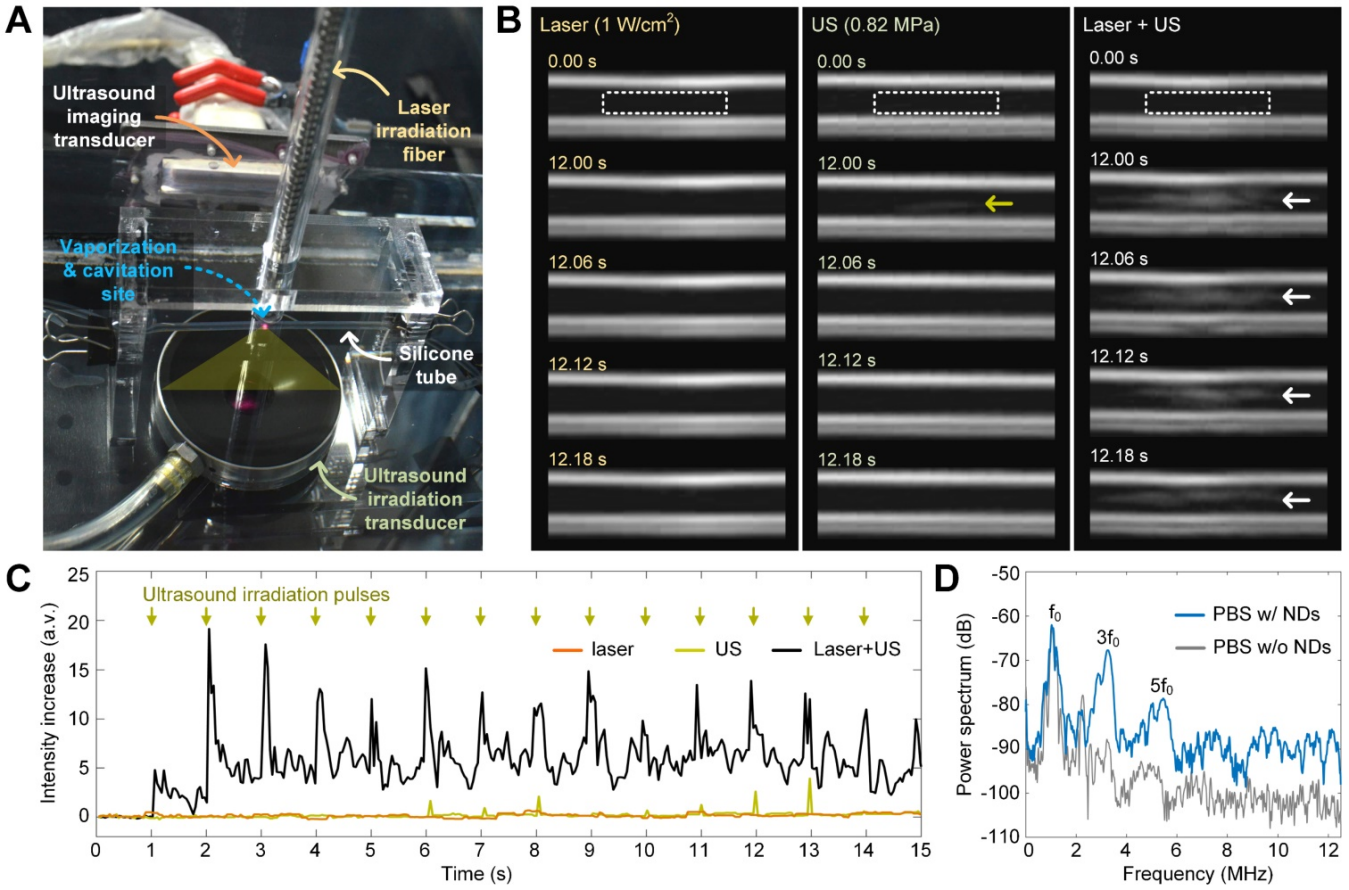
Nanodroplet vaporization and microbubble cavitation induced by OASI. (A) Experiment setup for real-time ultrasound imaging of nanodroplet vaporization inside a 1.5-mm-diameter silicone tube. (B) Ultrasound imaging sequences demonstrating the efficiency differences of nanodroplet vaporization induced by laser irradiation, ultrasound, or OASI. (C) Quantitative measurements of the intensity increases of echo signals reflected from the generated microbubbles. (D) Power spectra of the echo signals received with and without nanodroplets in PBS buffer during OASI. NDs: nanodroplets.
